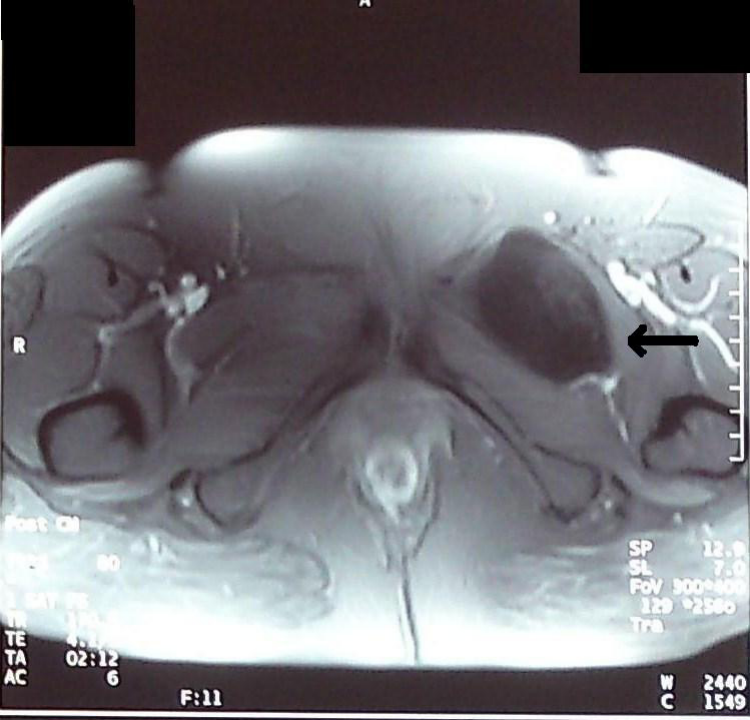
Axial T1-weighted MRI of left groin Intramuscular myxoma.
